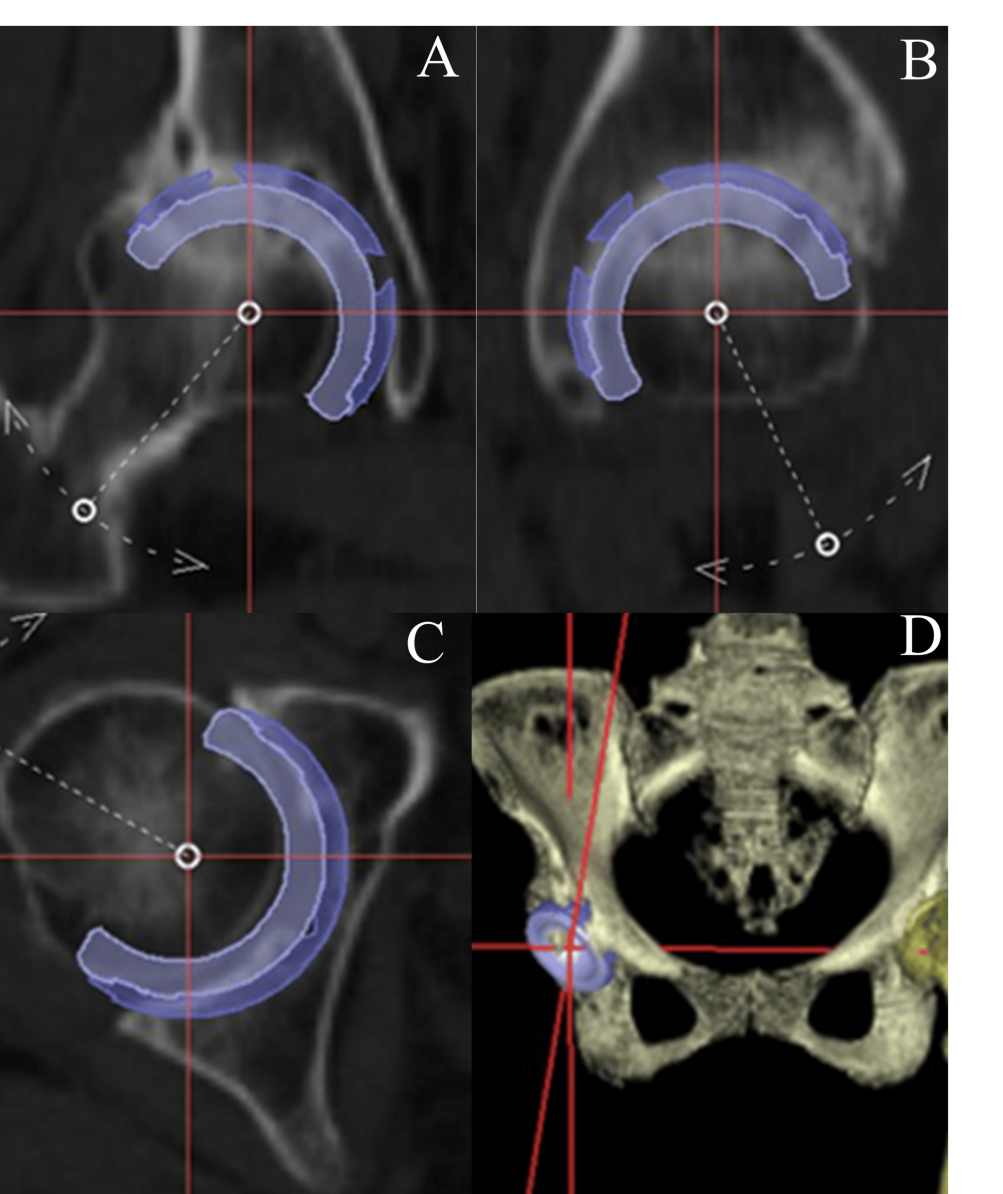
Extraction of coordinates for three-dimensional positioning of cups in preoperative planning. A: Longitudinal slice view B: Sagittal slice view C: Transverse slice view D: Three-dimensional CT
